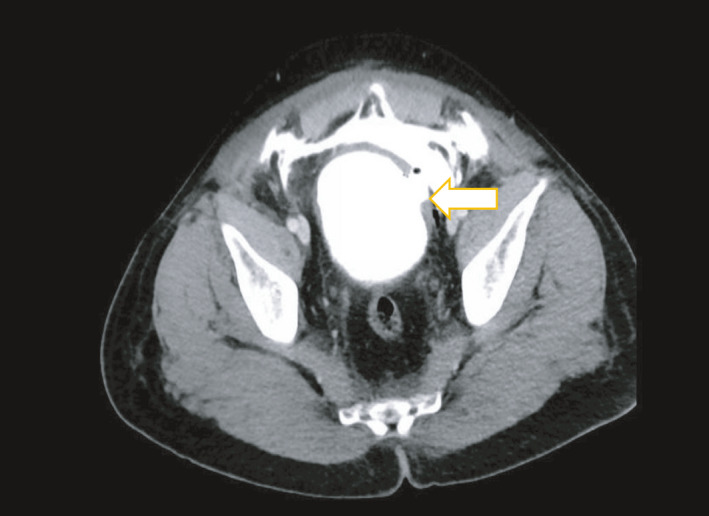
Abdominopelvic CT scan showed extravasation of contrast from the bladder to the pelvis through left lateral bladder wall defect
